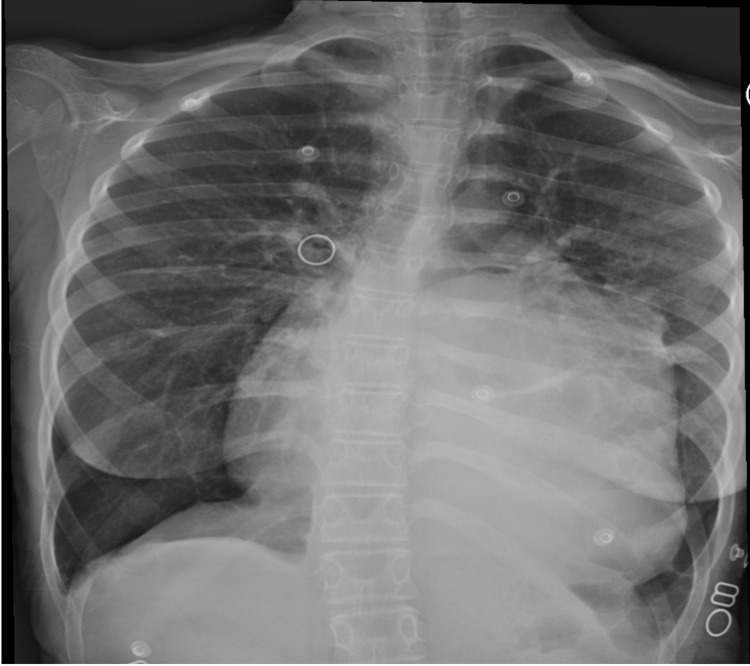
Chest x-ray revealed cardiac silhouette enlarged with hyperinflated lung and left retrocardiac airspace opacity with subsegmental atelectasis, as well as blunted bilateral costophrenic angles.
